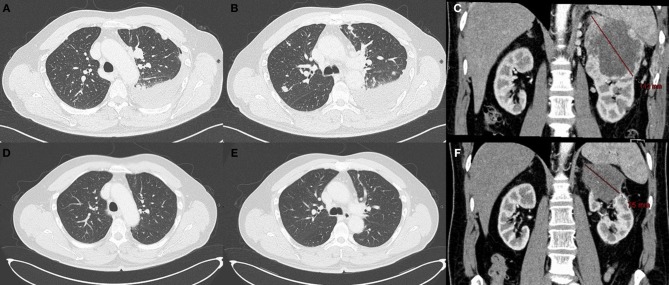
CT scan after sunitinib therapy and while under nivolumab Pulmonary metastasis (A,B) and renal lesion (C) after progression under sunitinib. Radiological complete response of the pulmonary metastasis (D,E) under nivolumab therapy at 6 months. The CT scan showed only a 75 mm mass on the left kidney (F).
